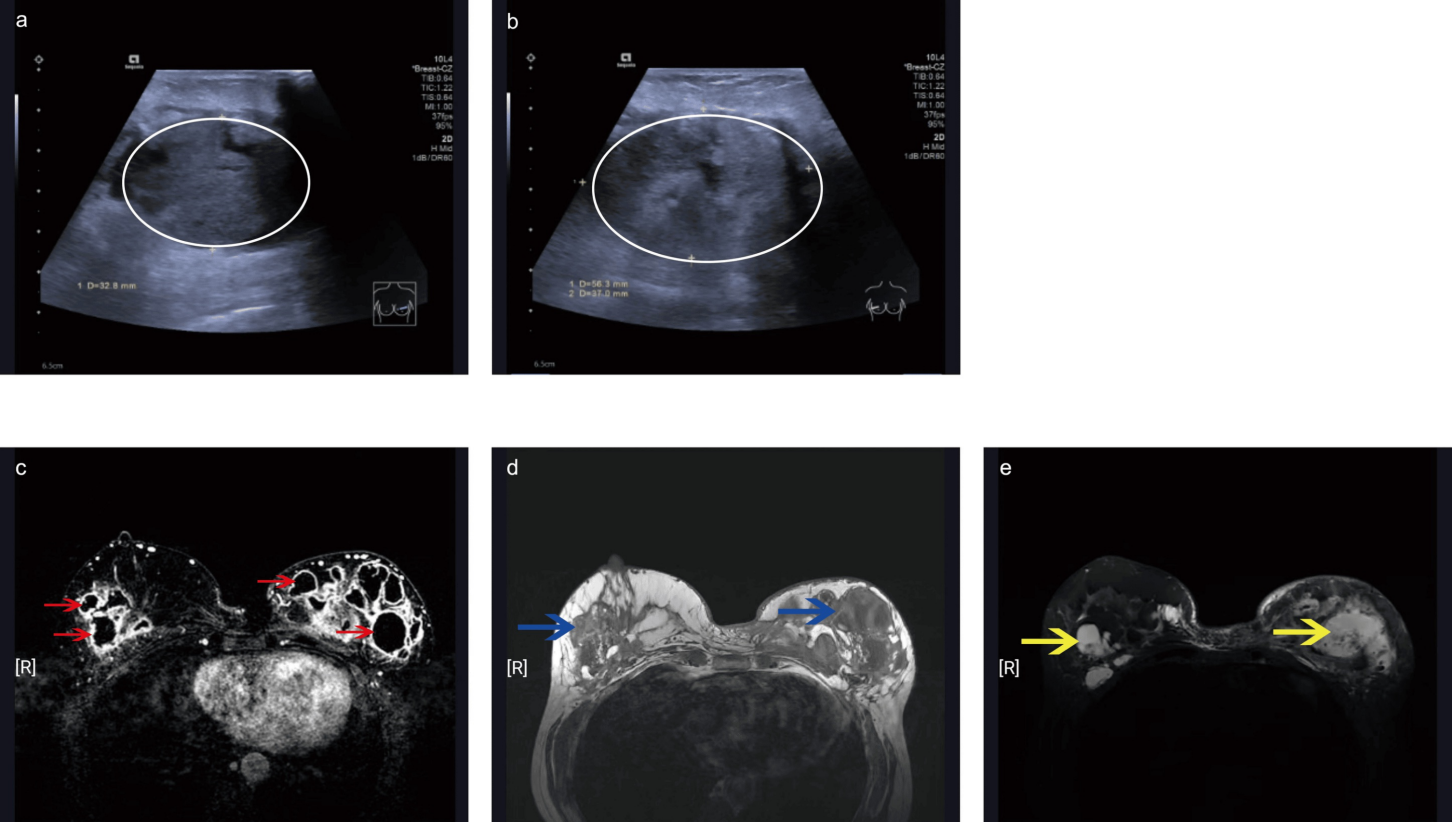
Ultrasonography. Several hypoechoic areas without a clear boundary in the retroglandular space of both breasts (a: left, b: right) suggest abscess formations (ellipse circle). Bilateral breast MRI. (c) Multiple ring-shaped enhancing foci (red arrows) without a clear boundary appear in the retroglandular space, and it was considered as abscess formation. Filler material showing low signal intensity (blue arrows) on fat-saturated T1-weighted (d) and high signal intensity (yellow arrows) on T2-weighted images (e). MRI: magnetic resonance imaging
